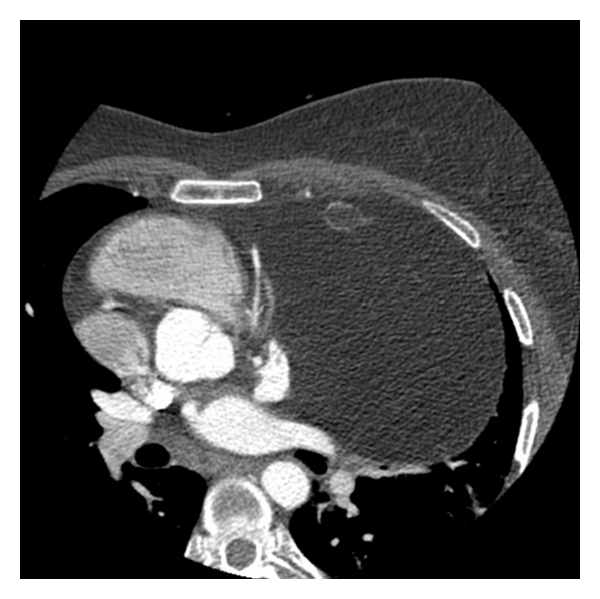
CT of the thorax with the left-sided lipomatous mass displacing the heart to the right hemithorax.
